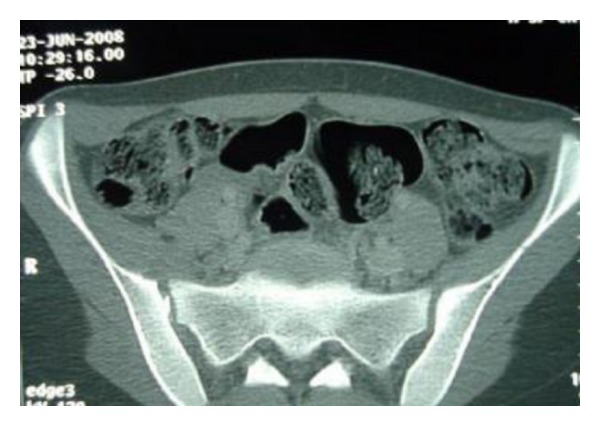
CT of the pelvis revealed apparent widening of the right sacroiliac joint with infiltration, edema of the soft tissue surrounding the joint, and presence of microabscess in the right iliacus muscle.
